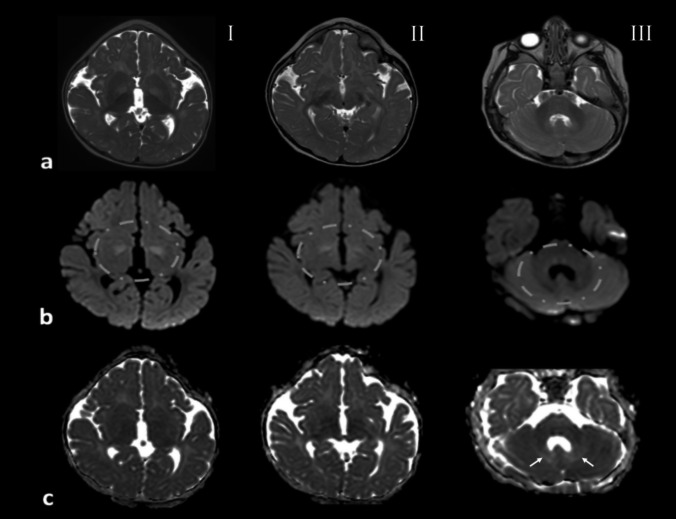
Brain magnetic resonance imaging of a 9-month-old boy with developmental and epileptic encephalopathy, trigonocephaly due to metopic suture synostosis, and a pathogenic *POLR2A* variant, treated with Sabril® (125 mg/kg/day). (*a, b*) Axial T2-weighted turbo spin-echo (*a*) and axial diffusion-weighted (*b*) images without contrast administration demonstrate bilateral and symmetrical hyperintensity (*circles*) involving the globi pallidi (*I*), subthalamic nuclei (*II*), and central tegmental tracts (*III*). There is mild hyperintensity of the dentate nuclei (*arrows*). (*c*) Corresponding axial apparent diffusion coefficient images demonstrate restricted diffusion of all abnormal sites except for the dentate nuclei (*arrows*)
